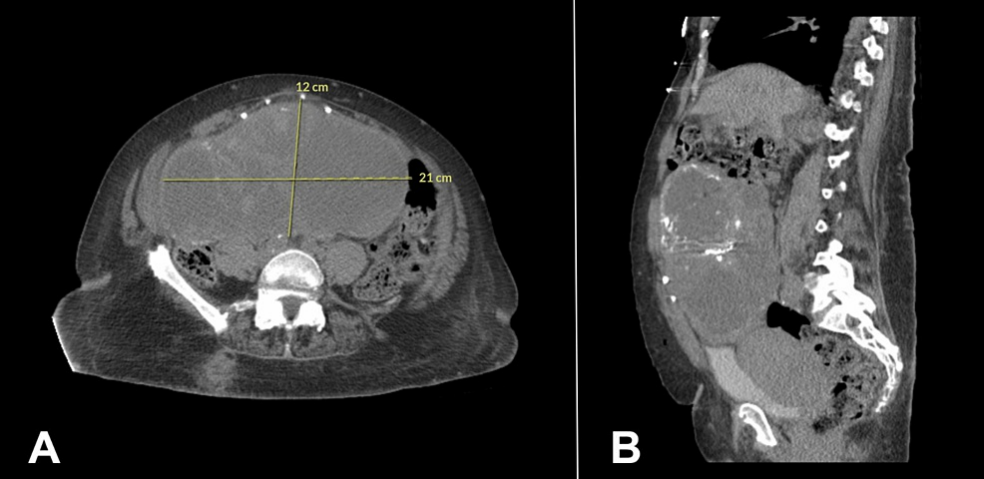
CT of the abdomen and pelvis without contrast Large multiloculated cystic mass with areas of calcification within and multiple septations extending from the left adnexa to the upper abdomen shown on the transverse (A) and sagittal (B) planes CT: computed tomography
